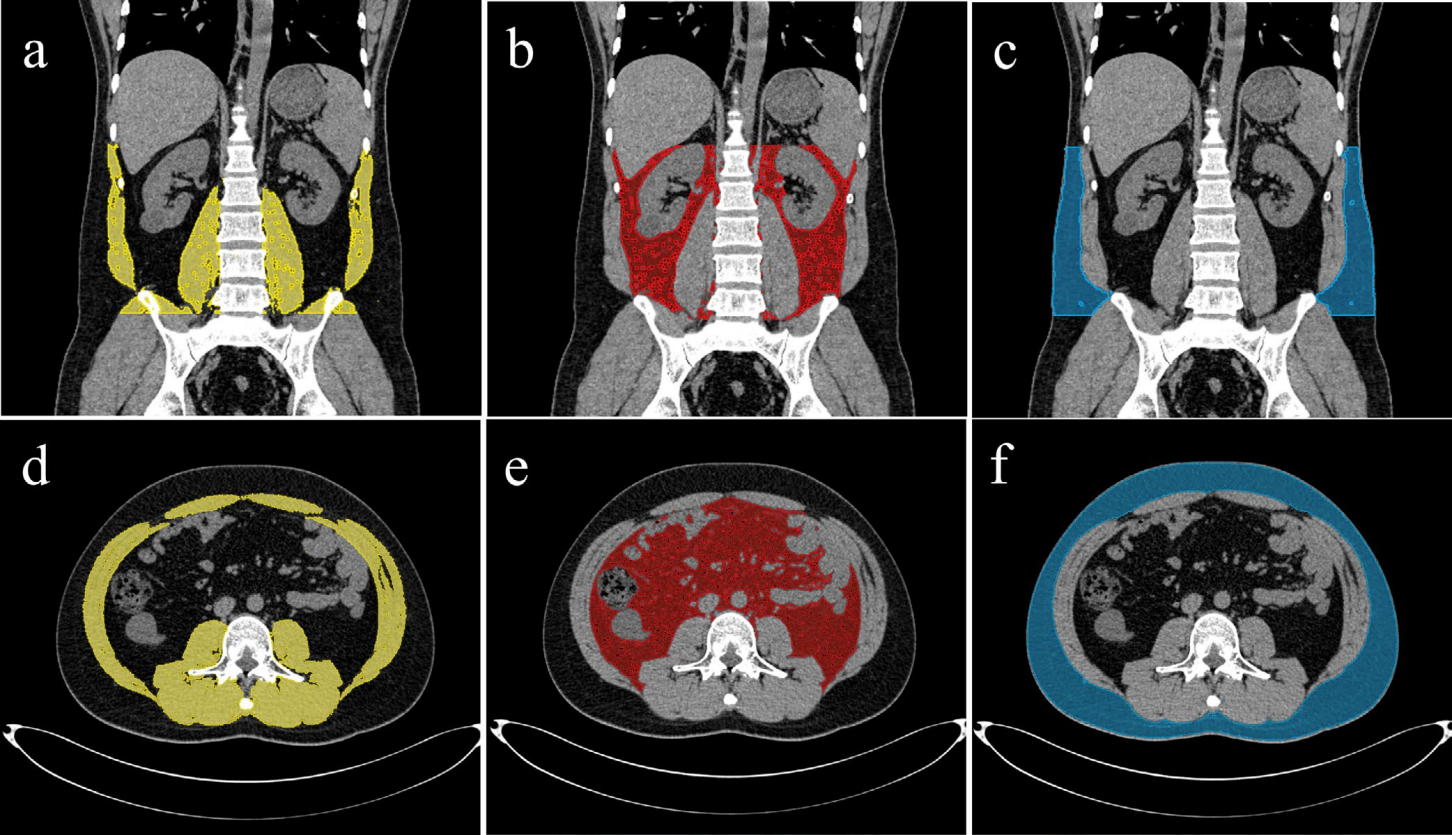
Assessment of abdominal fat and muscle compositions using 3D Slicer software. Images (a, b, and c) display axial CT scans, highlighting the measured volumes and densities. Images (d, e, and f) depict axial CT scans, emphasizing the measured areas and densities, with skeletal muscle marked in yellow, visceral adipose tissue in red, and subcutaneous adipose tissue in blue.
